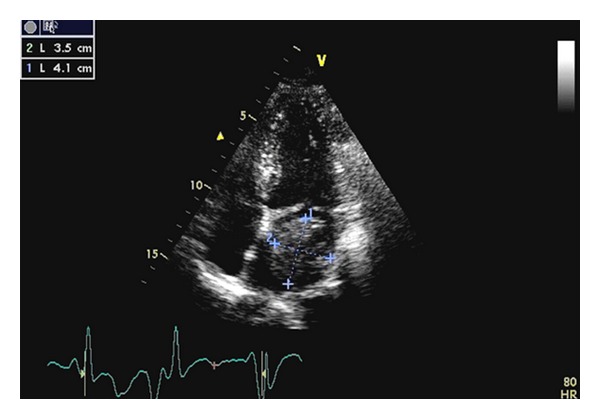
Transthoracic echocardiogram showing left atrial mass obstructing left ventricular outlet tract and originating from left superior pulmonary vein.
